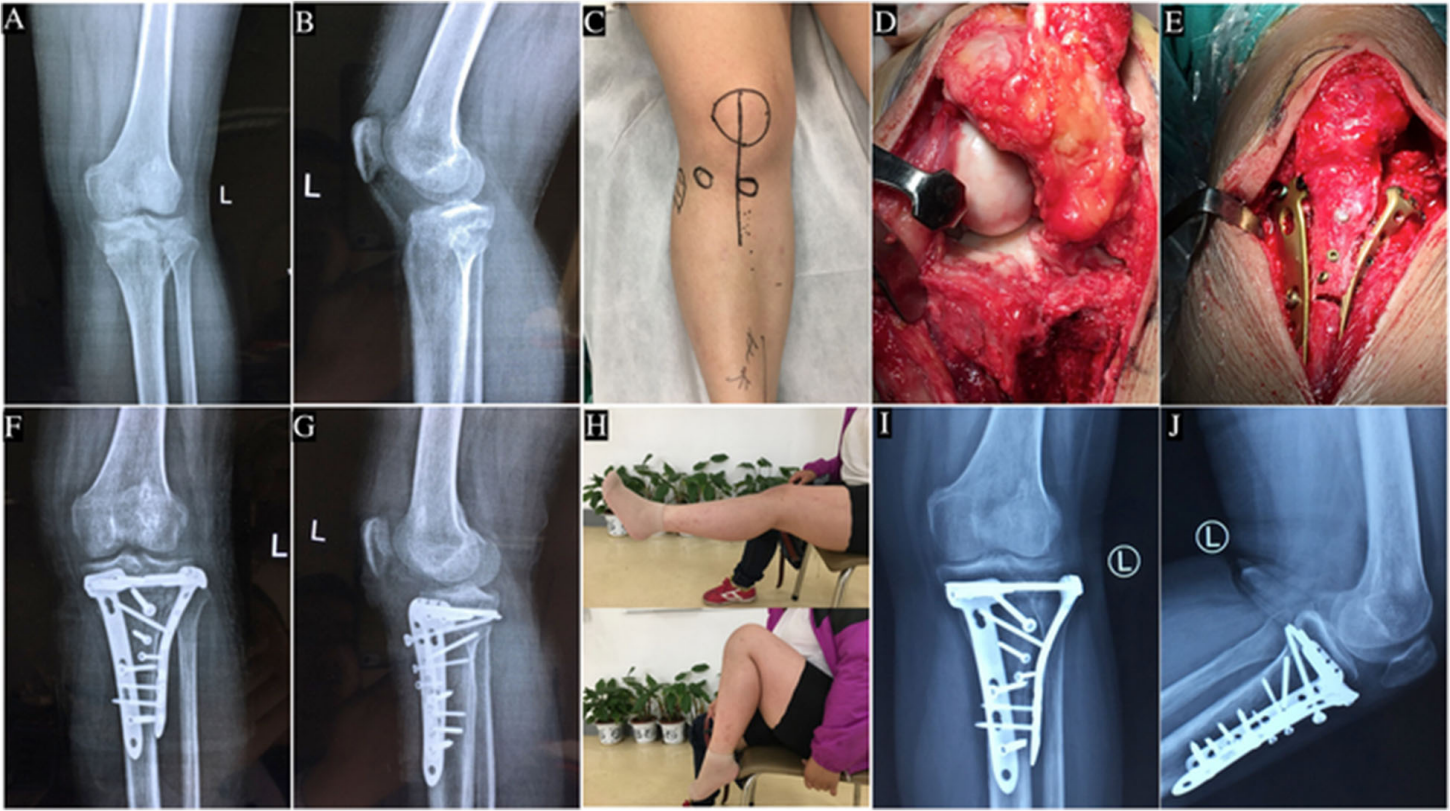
A 26-year-old woman with an old hyperextension bicondylar tibial plateau fracture treated with modified anterior midline incision and tibial tuberosity osteotomy. a,b Preoperative anteroposterior view and lateral view of X-ray. c Modified anterior midline incision. d The whole Tiankeng-like collapsed fragment can be exposed after the osteotomized tibial tuberosity is retracted proximally. e Intraoperative photograph shows the fracture was fixed with double plates and osteotomized tibial tuberosity was fixed with 3cortical screws. f,g Postoperative anteroposterior view and lateral view of X-ray. h Range of motion of the knee is close to normal at the 3.5-month follow up postoperatively. i,j Anteroposterior view and lateral view of X-ray show no reduction loss occurred and the fracture has healed 3.5 months after surgery
